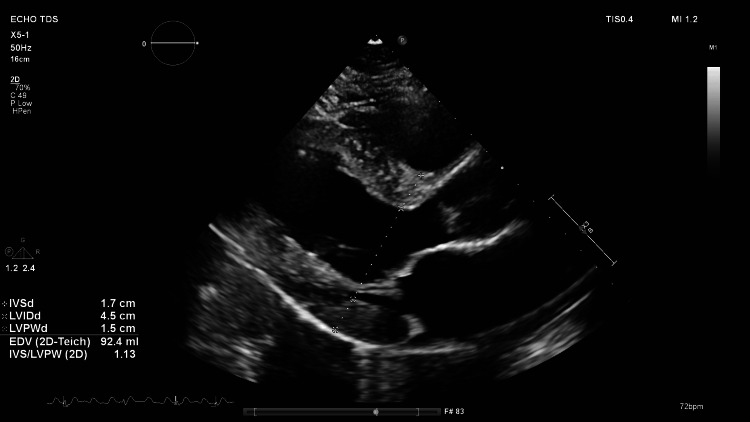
Echocardiogram showing severe left ventricular hypertrophy with bright and echogenic endocardium from amyloid infiltration
